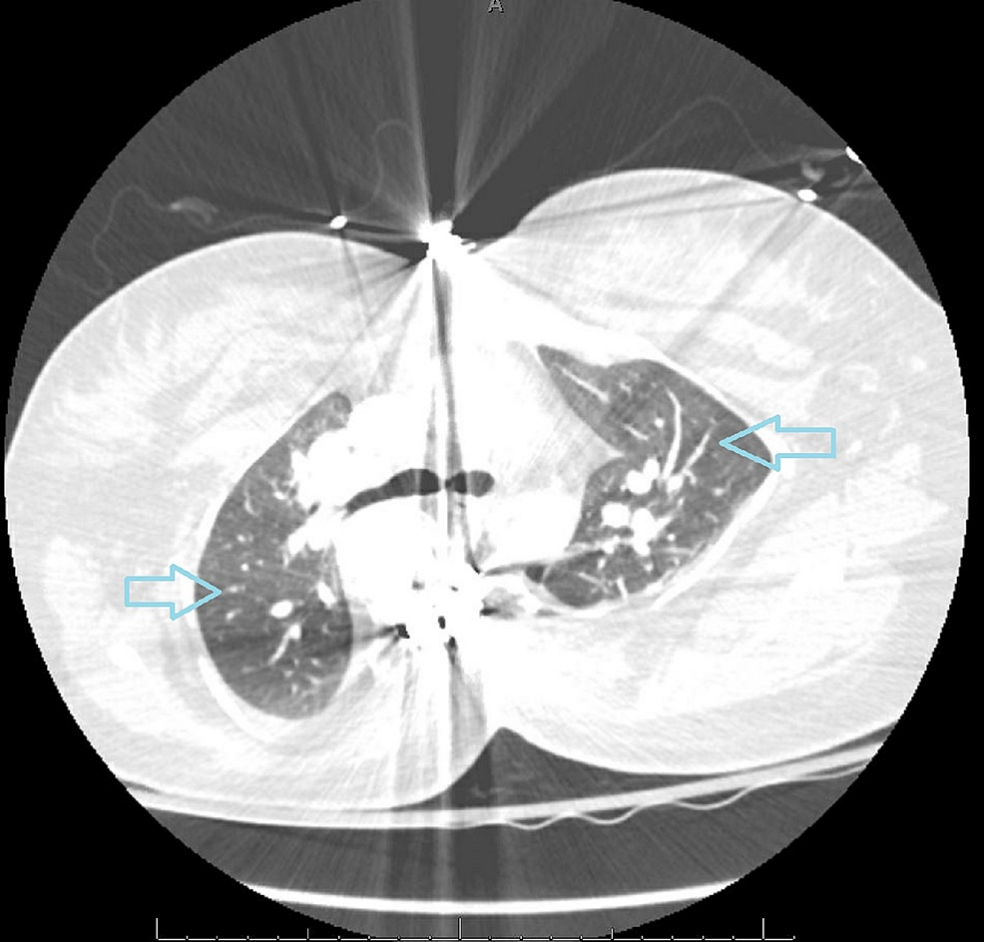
CT of the chest, lung window. Blue arrows: evidence of restrictive lung disease with decreased lung volumes. CT: computed tomography
